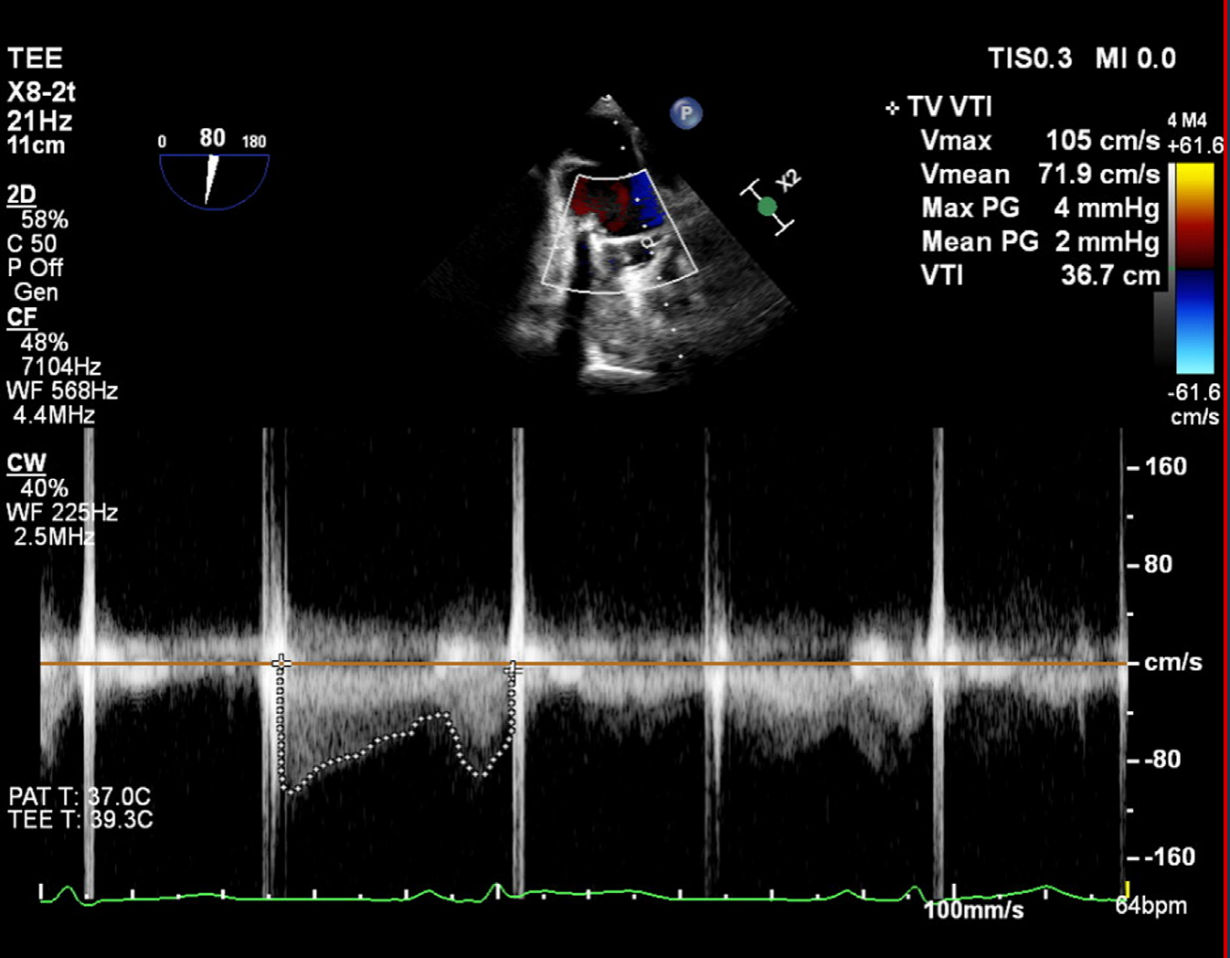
Pulsed Wave Doppler Across Mechanical Tricuspid Valve Replacement on Transesophageal Echocardiography, 48 h Post-Thrombolysis
